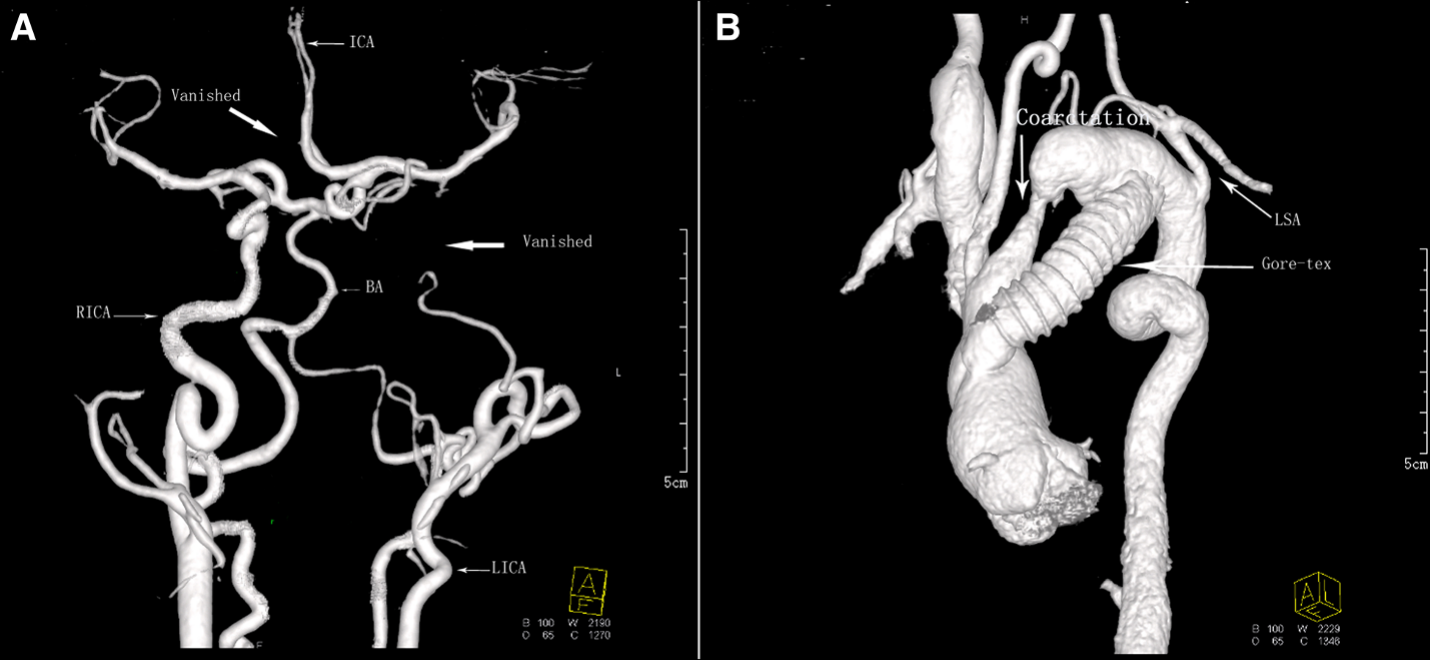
CTA showed multivessel distortion and malformation. A: Cerebrovascular CTA showed the distal LICA and the origin of the RACA were vanished, the BA supplied blood for the whole left brain. B: A 13-mm Gore-tex tube was implanted as a bypass between the ascending aorta and the descending aorta.
